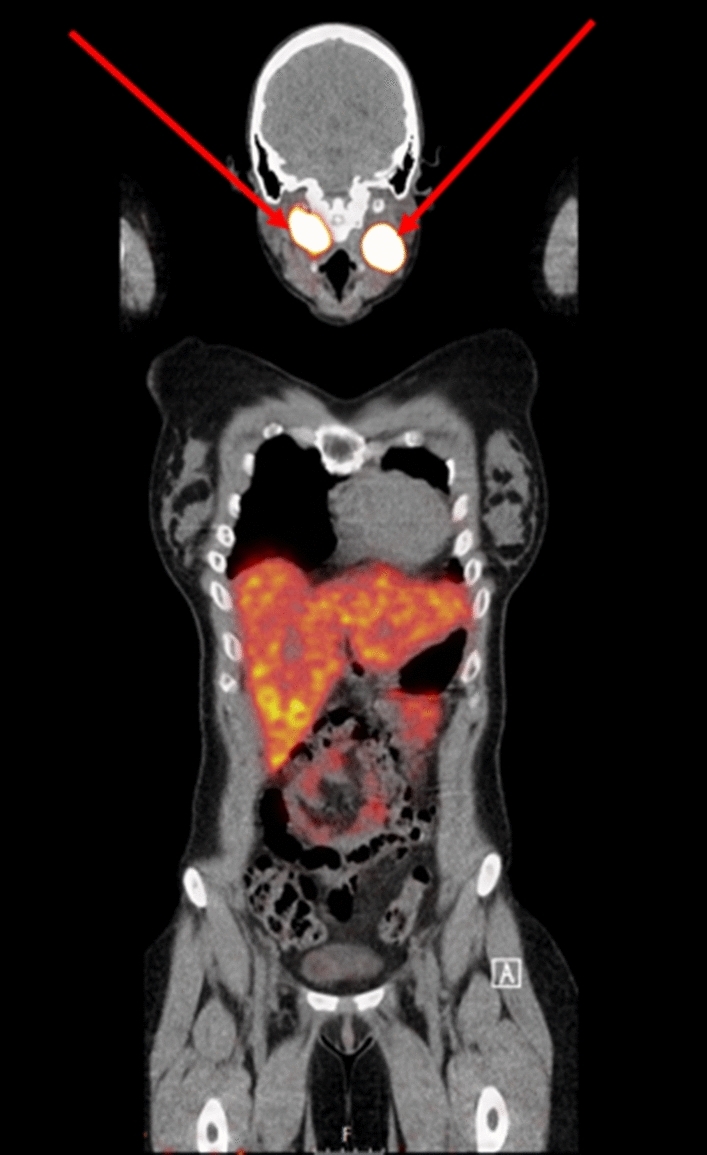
68 Ga-DOTATATE PET-CT scan demonstrating increased uptake in a right-sided vagal body tumour and a left-sided carotid body tumour in patient 2
